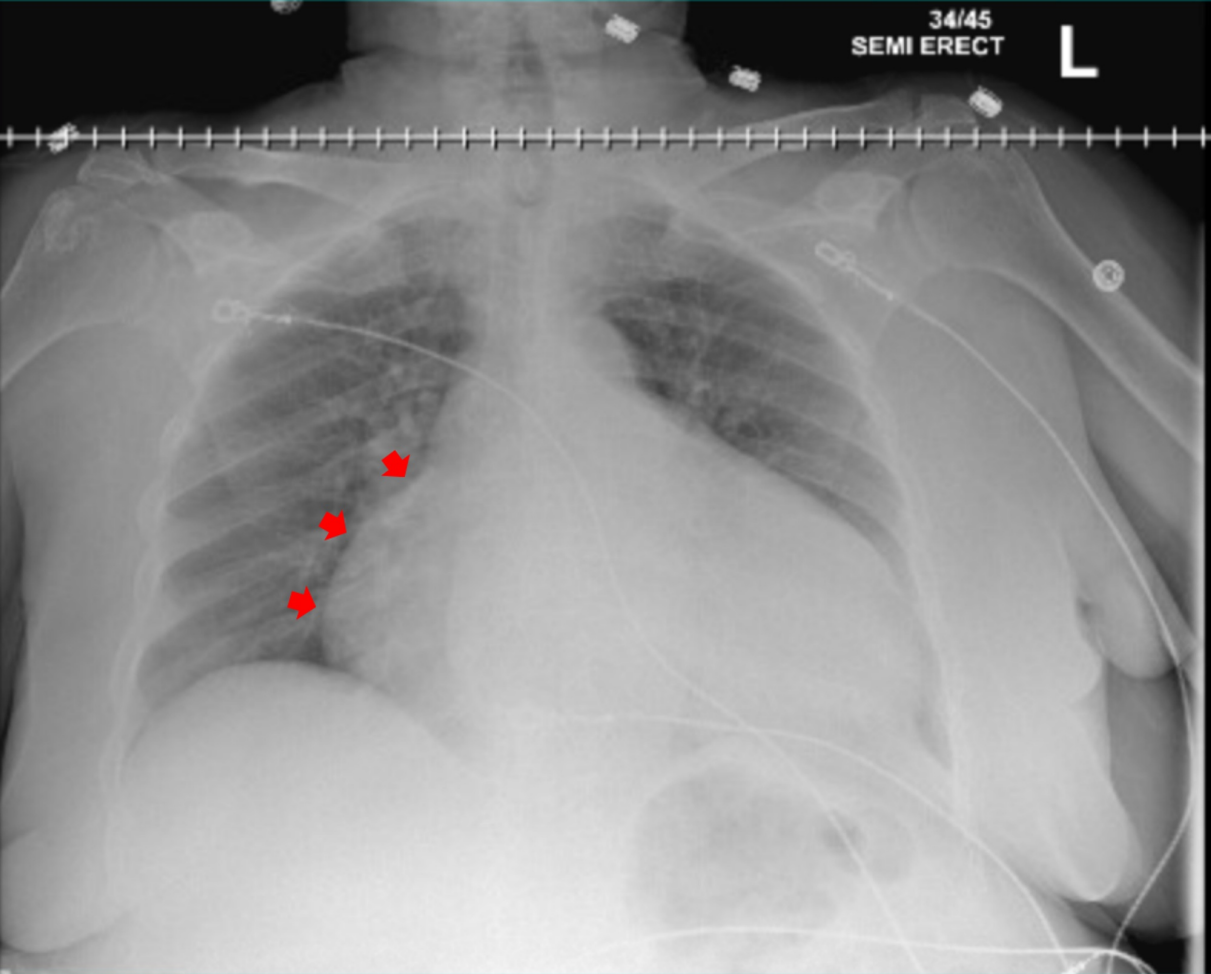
One-view upright anterior-posterior chest radiograph The image shows cardiomegaly with large cardiac silhouette, and low lung volumes. Red arrows highlight enlarged right ventricle
